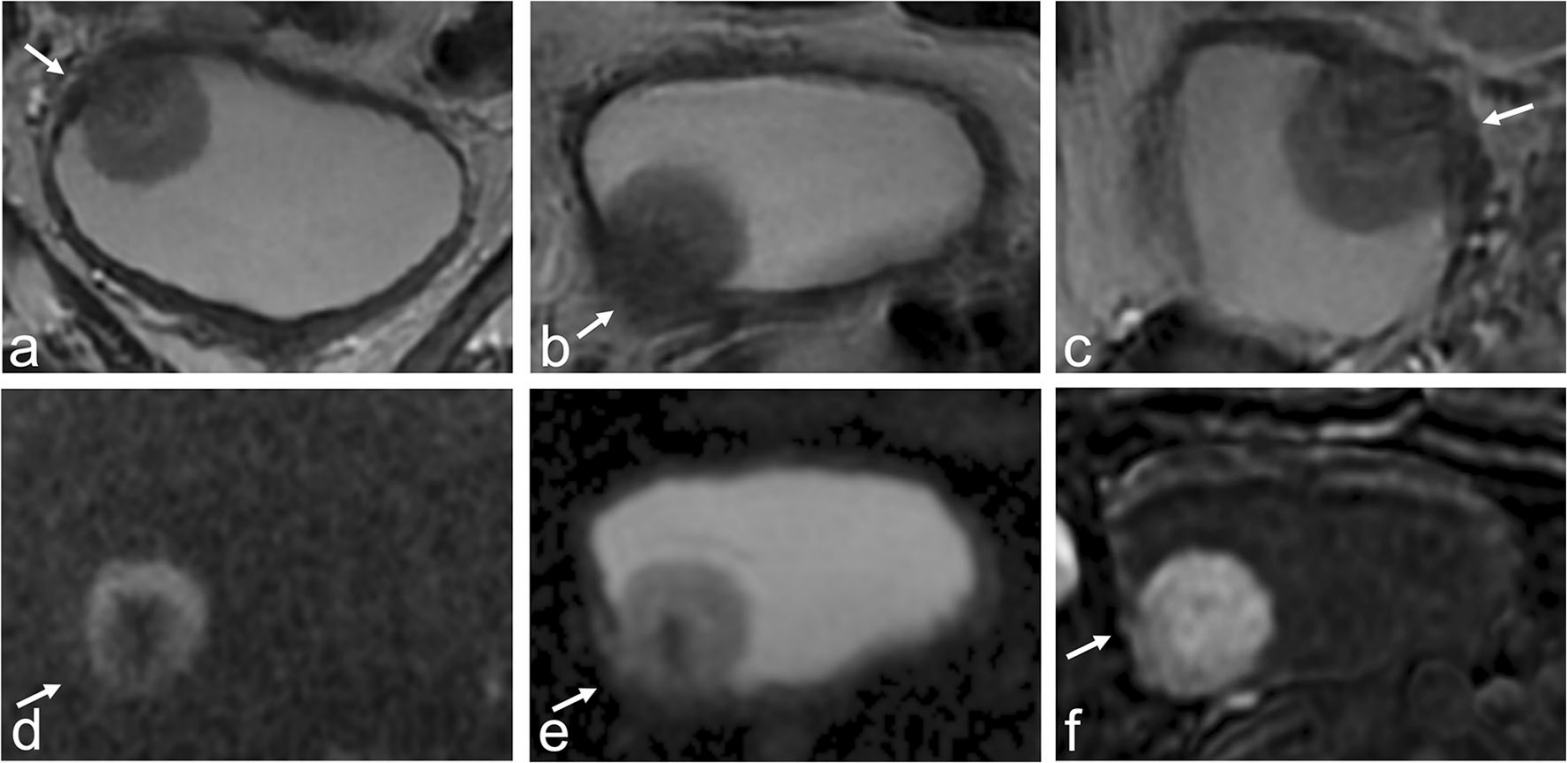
Case example of a 67-year-old male. a Coronal T2WI showing a pedunculated bladder tumor at the bladder dome extending to the right lateral wall with a clearly uninterrupted muscularis propria layer (arrow); b axial T2WI showing an apparently interrupted muscularis propria (arrow); c sagittal T2WI showing an equivocal alteration of the muscularis propria (arrow); d, e DWI and ADC map showing no interruption of the muscularis propria layer (arrows), and the “inchworm sign”, which is usually indicative, as in this case, of NMIBC; f DCE MRI showing the integrity of the *muscularis propria* layer and the inner layer sign (arrow), both indicative of NMIBC. The images were incorrectly scored as a VI-RADS 3 and 4 by the inexperienced readers during the first interpretation batch, probably due to the non-optimal quality acquisition; however, MRI was correctly scored with an overall VI-RADS 2 in batch 5, given the higher reader experience. T stage after TURBT identified HG-T1 urothelial carcinoma. T2WI, T2-weighted imaging; VI-RADS, Vesical Imaging-Reporting and Data System; DWI, diffusion-weighted imaging; ADC, apparent diffusion coefficient; DCE, dynamic contrast-enhanced; NMIBC, non-muscle-invasive bladder cancer; TURBT, trans-urethral resection of bladder tumor; HG, high-grade
